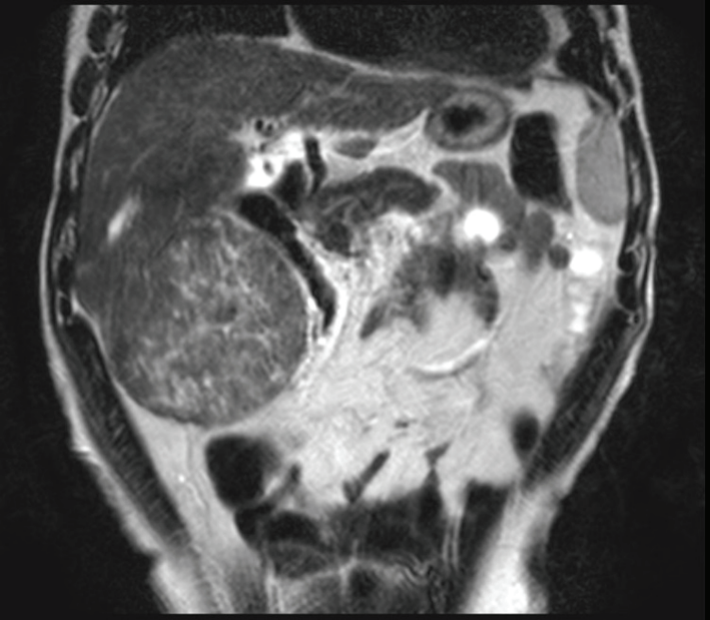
Abdominal contrasted MRI, T2-weighted, showing the tumor. It presented close proximity to the right kidney and the hepatic flexure of the colon.
